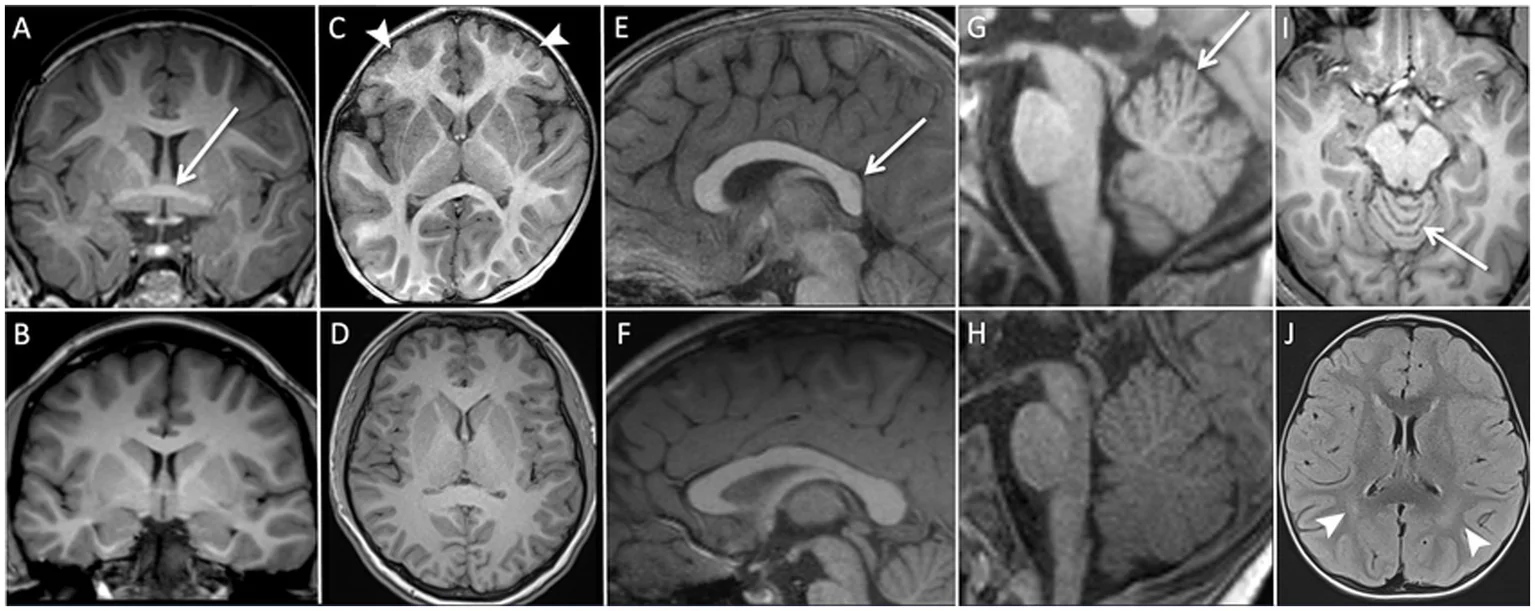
Brain MRI non-specific abnormalities. (A) Coronal T1-weighted sequence: thickened appearance of the anterior commissure (white arrow). (B) Coronal T1-weighted sequence: normal appearance of the anterior commissure. (C) Axial T1-weighted sequence: subtle triangular shape of the anterior cranial vault with frontal lobe hypoplasia (white arrow heads). (D) Axial T1-weighted sequence: normal appearance of cranial vault and frontal lobes. (E) Sagittal T1-weighted sequence: short corpus callosum (white arrow). (F) Sagittal T1-weighted sequence: normal corpus callosum. (G) Sagittal T1-weighted sequence: widening of the superior vermian sulci (white arrow). (H) Sagittal T1-weighted sequence: normal appearance of the cerebellar vermis. (I) Axial T1-weighted sequence: widening of the superior vermian sulci (white arrow). (J) Axial FLAIR sequence: periventricular leukopathy (white arrow heads).
